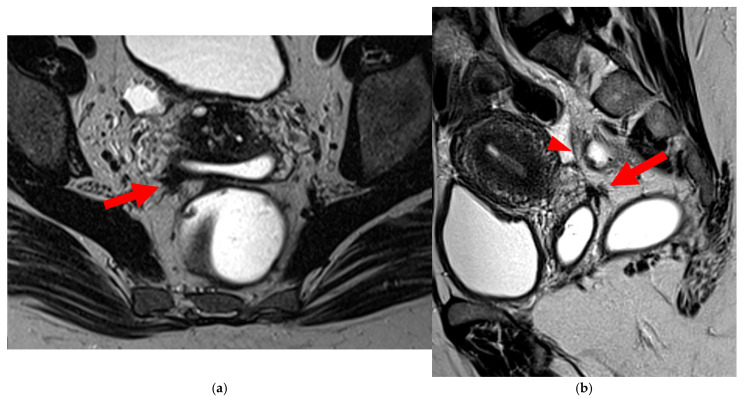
Pelvic MRI scan of a patient with a HTD type 6 right USL. (a,b) Axial (a) and sagittal (b) T2WI show a spiculated nodular hypointense lesion (*arrows*) located in the right cardinal ligament (a.k.a. Mackenrodt ligament), affecting the uterovaginal nerve plexus, a component of the inferior hypogastric plexus. Note the steep vertical orientation of the right proximal USL (*arrowhead*), which is reclassified from type 3B to type 6 due to this so-called “visceral” involvement.
